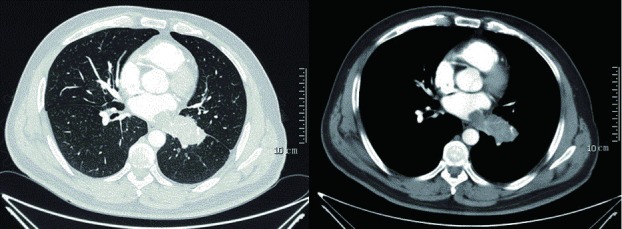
病例1术前胸部增强CT Preoperative chest CT image of case No.1
